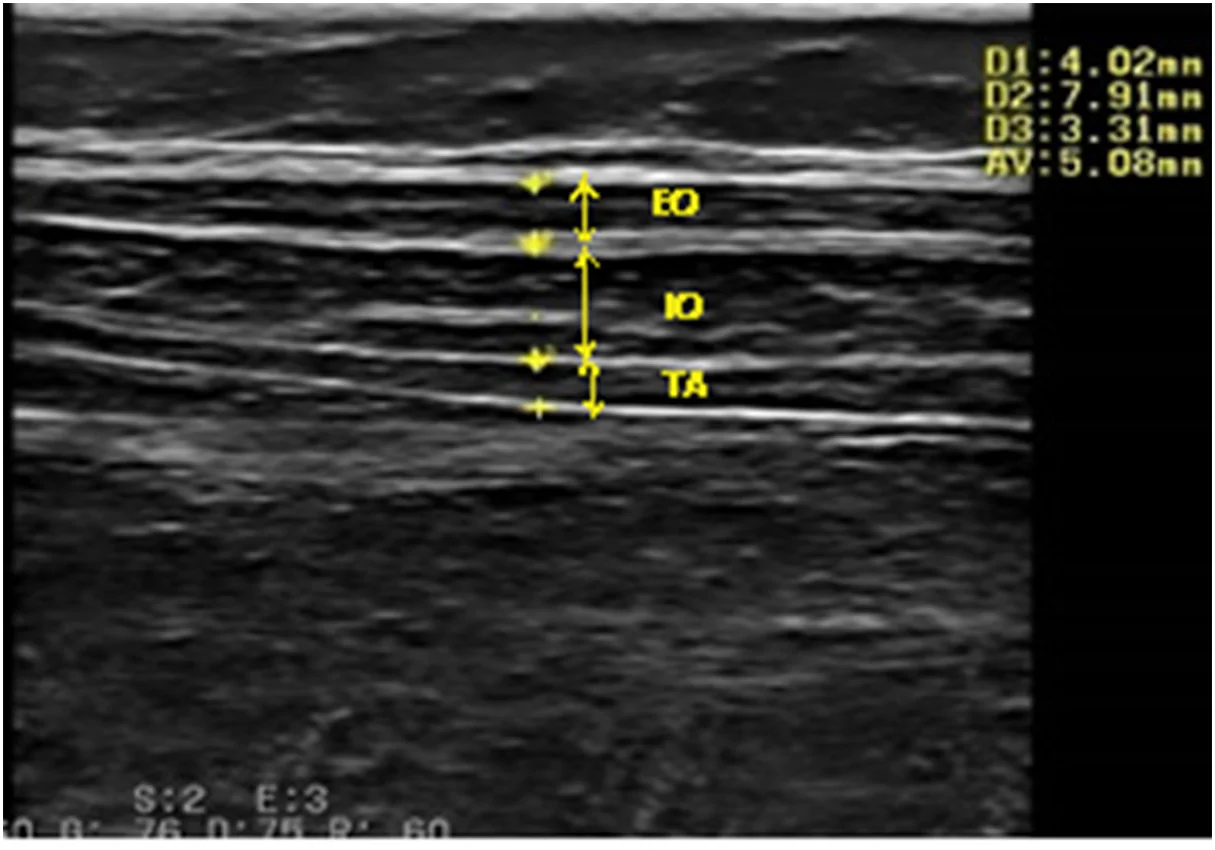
Ultrasound image of abdominal muscles in contracted state. Created by the authors. Published under CC BY 4.0.
